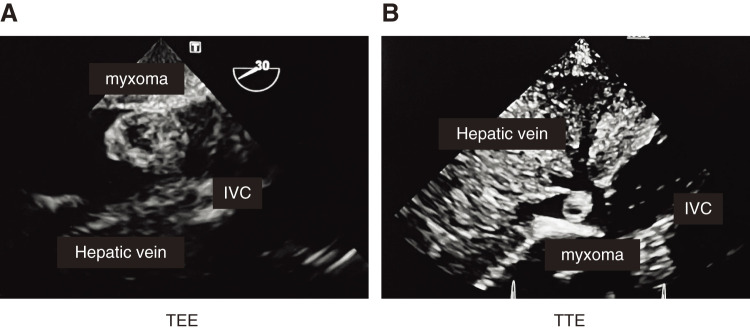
Fig. 1 The mass showed low echogenicity and hypovascular characteristics. (A) Transthoracic echocardiography. (B) Transesophageal echocardiography.
